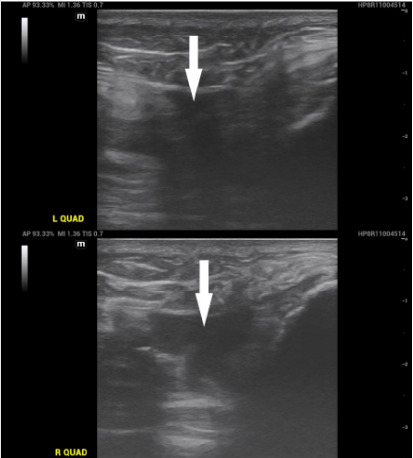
Point-of-care ultrasound of the left and right suprapatellar regions in sagittal plane demonstrating disruption in the bilateral quadriceps tendon fibers with adjacent anechoic fluid collections likely representing hematomas (arrows). *L QUAD*, left quadriceps; *R QUAD*, right quadriceps.
